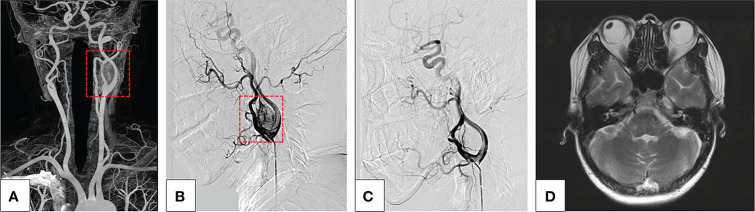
(A–D) A typical case of a severe complication after endovascular treatment of CBT. (A) Maximum-intensity-projection CTA images show a left CBT, Shamblin II category (red box). (B) The lateral view of DSA image before embolization of the feeding arteries shows a highly vascularized CBT (red box), and the embolization was carried out after superselection into the feeding vessel with 100–300 μm PVA particle. Almost all blood supply was obstructed during the embolization procedure. During the procedure, the patient suffered a sudden onset of left visual loss. (C) DSA manifested that the blood supply of CBT was significantly reduced and no abnormalities occurred in the main left ophthalmic artery. (D) MRI did not show any abnormal signs in the left eye.
